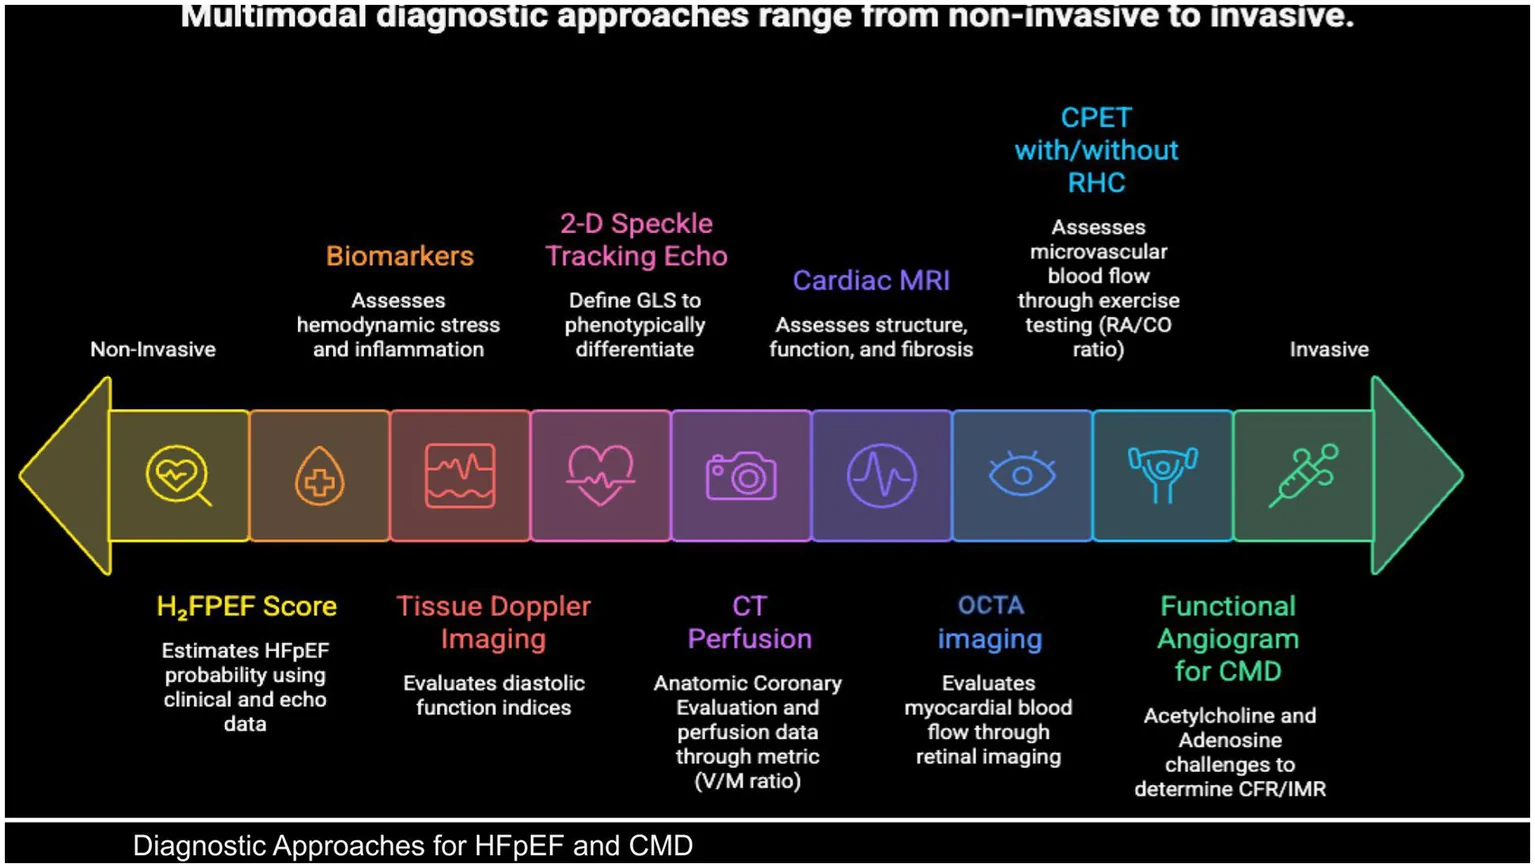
Multimodal diagnostic approaches to HFpEF and CMD, ranging from non-invasive to invasive. Spectrum of diagnostic modalities used to evaluate HFpEF and coronary microvascular dysfunction, arranged along a continuum from non-invasive (left) to invasive (right). Modalities shown are the H₂FPEF score, which estimates HFpEF probability from clinical and echocardiographic data; biomarkers, which assess haemodynamic stress and inflammation; tissue Doppler imaging, which evaluates diastolic function indices; two-dimensional speckle-tracking echocardiography, which defines global longitudinal strain to phenotypically differentiate; computed tomography perfusion, which provides anatomic coronary evaluation and perfusion data via the volume-to-mass ratio; cardiac magnetic resonance imaging, which assesses structure, function, and fibrosis; OCTA imaging, which evaluates myocardial blood flow through retinal microvascular imaging; cardiopulmonary exercise testing with or without right heart catheterization, which assesses microvascular blood flow through exercise testing; and functional angiography, which uses acetylcholine and adenosine challenge to determine coronary flow reserve and the index of microvascular resistance. CFR, coronary flow reserve; CMD, coronary microvascular dysfunction; GLS, global longitudinal strain; IMR, index of microvascular resistance; OCTA, optical coherence tomography angiography; RHC, right heart catheterization.
